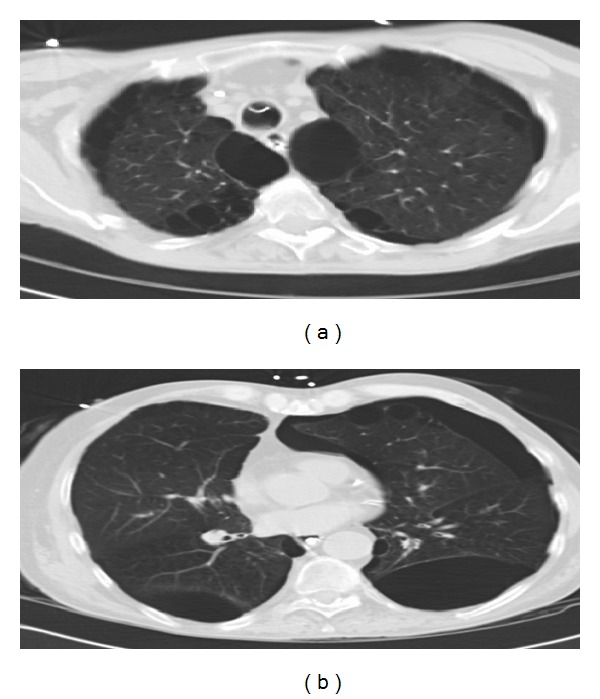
Chest CT axial view showing bilateral upper lobe (a) and lower lobe (b) bullae compressing surrounding relatively normal lung parenchyma.
